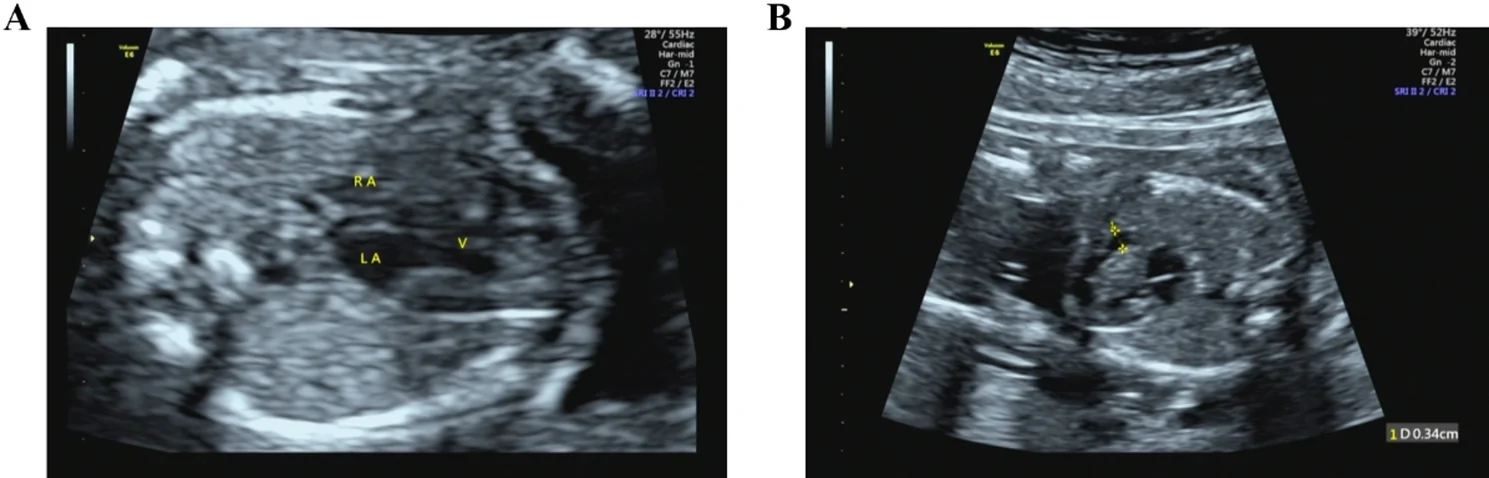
Fetal echocardiography at 19 weeks. (A) Functional single ventricle and atrioventricular valve (cardio-thoracic index<0.33). (B) The left and right ventricles were connected by a common arterial trunk and the effusion was seen in the pericardial cavity (3.4 mm)
